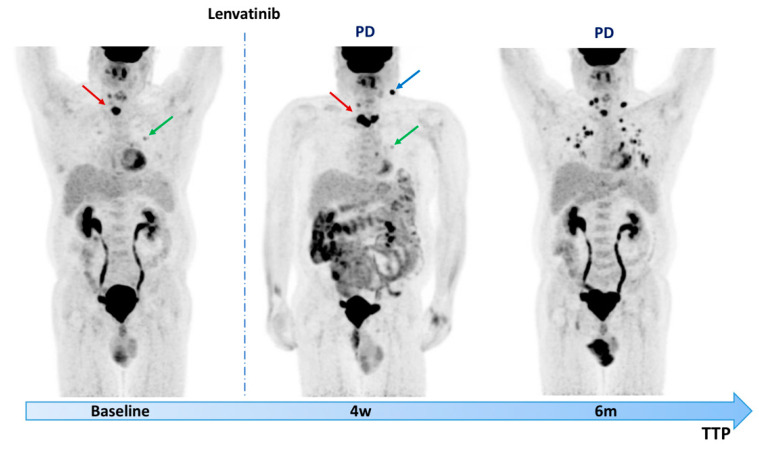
A 63-year-old man with metastatic RAI-R well-differentiated follicular cell thyroid cancer underwent [^18^F]FDG PET/CT before starting lenvatinib therapy. Baseline PET showed an intense FDG uptake in the paratracheal region, corresponding to locoregional disease and moderate uptake in the bilateral pulmonary field. The patient then underwent reevaluation after 28 days from the beginning of MKI therapy, which showed disease progression with the appearance of a metastatic submandibular lymph node. After surgical lymph node resection, the patient continued lenvatinib therapy with disease progression 6 months later. PD = progression disease; w = week; m = month; TTP = time to tumor progression; RAI-R = refractory radioactive iodine; [^18^F]FDG PET/CT = 2-deoxy-2-[^18^F]fluoro-D-glucose positron emission tomography/computed tomography; MKIs = multi-kinase inhibitors.
